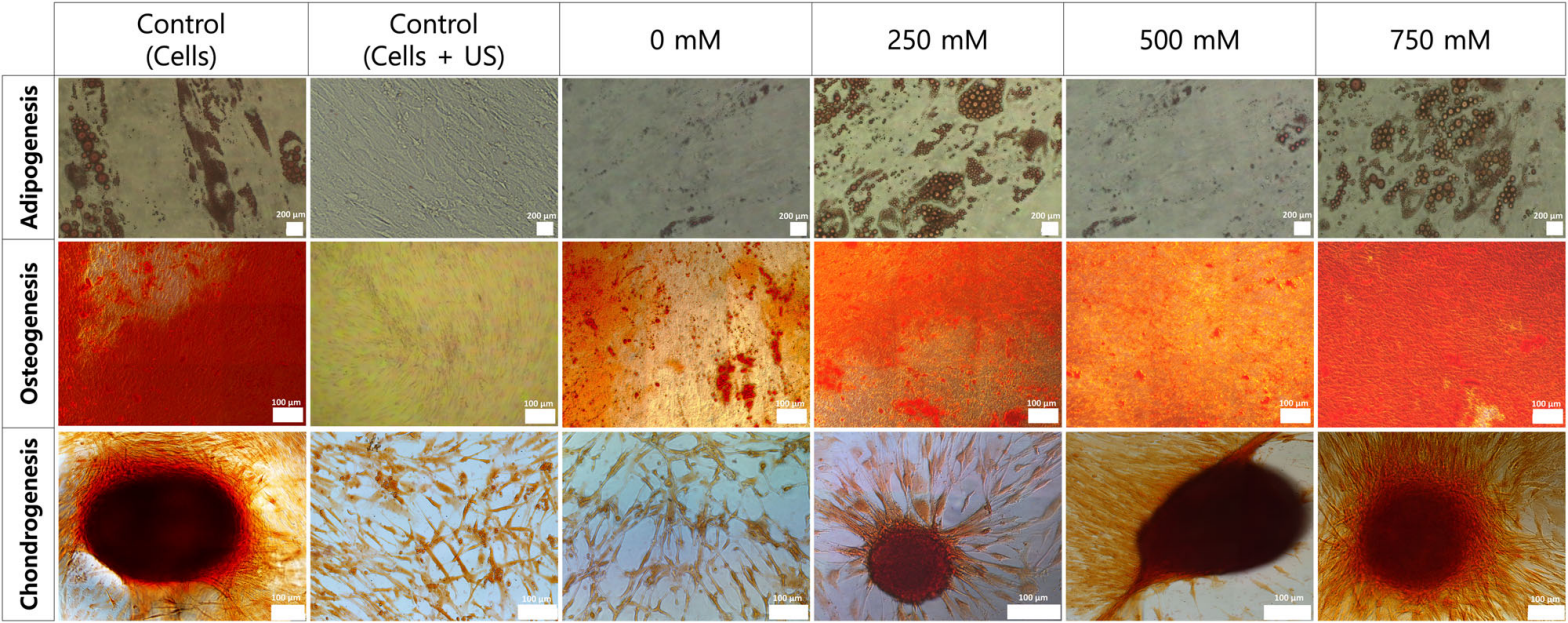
Cell pluripotency post-cryopreservation depicting sample images of cells treated with a range of trehalose concentrations (0–750 mM) delivered via UMT alongside controls. A control of MSCs not exposed to ultrasonication nor treated with trehalose (Control (cells)) and a control of cells only exposed to ultrasonication (0.25 MPa, 5.0 min; control (cells + US)) but not treated with trehalose were used. Controls were cryopreserved in 10% DSMO + 90% FBS to show the difference between DMSO and trehalose as CPA. During the differentiation assay, positive control cells are treated with the differentiation medium while negative controls are given normal medium: DMEM + 10% FBS. Scale bars: 200 μm (adipogenesis), 100 μm (osteogenesis), or 100 μm (chondrogenesis).
